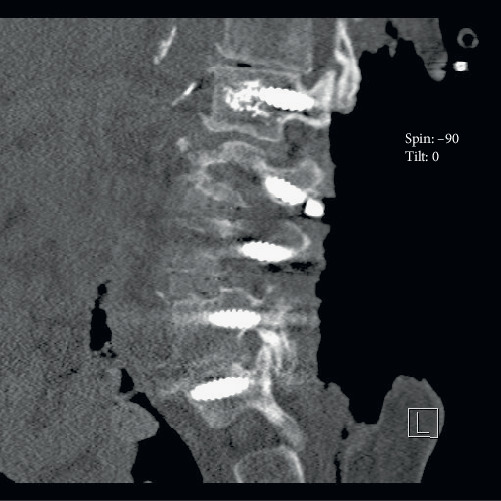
CT showing the placement of hardware prior to closing.
